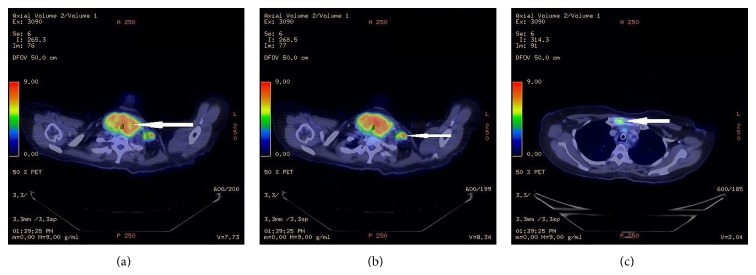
PET-CT scan showing (a) hypermetabolic enlarged thyroid gland, (b) hypermetabolic left cervical lymph node, and (c) hypermetabolic focus in right side of manubrium (indicated by arrows).
